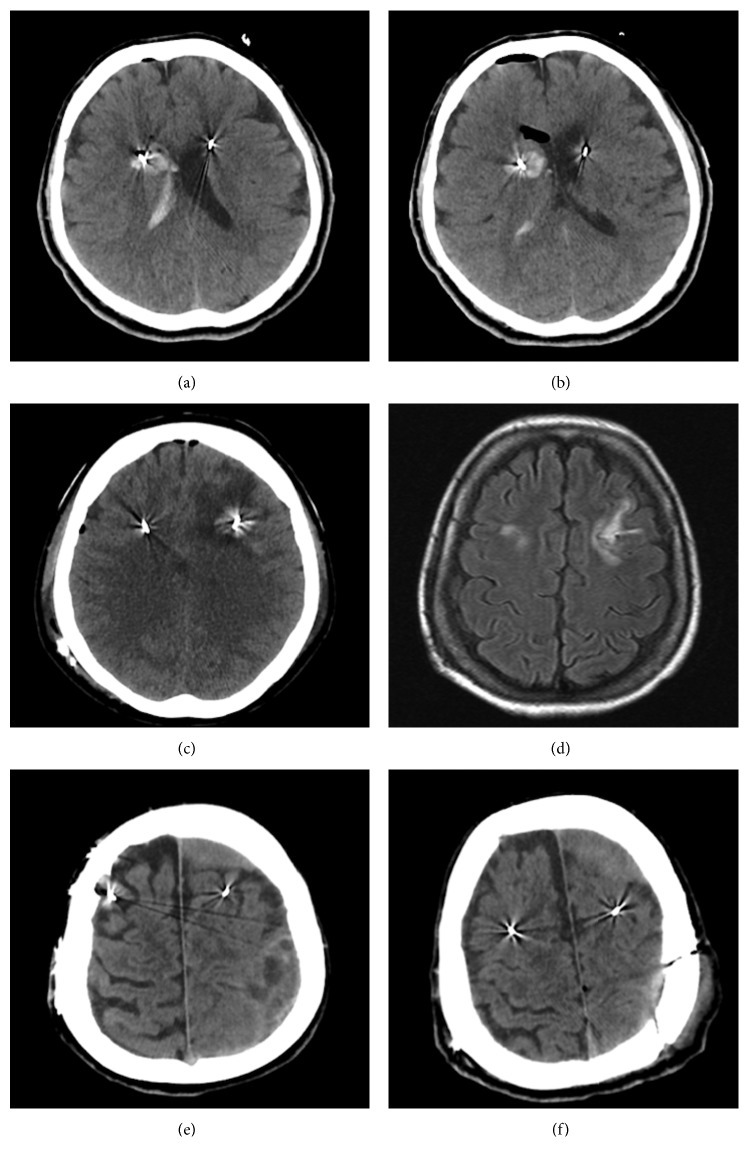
Operative complications due to deep brain stimulation surgery. (a, b) Right caudate hematoma with intraventricular hemorrhage. (c) Venous hemorrhagic infarction around the left-sided DBS lead; (d) 6-month follow-up FLAIR MRI. (e, f) Pre- and postoperative images of the left-sided chronic subdural hematoma over the parietal cortex. Note that the frontal component of the cSDH was left intact to avoid iatrogenic injury to the lead.
